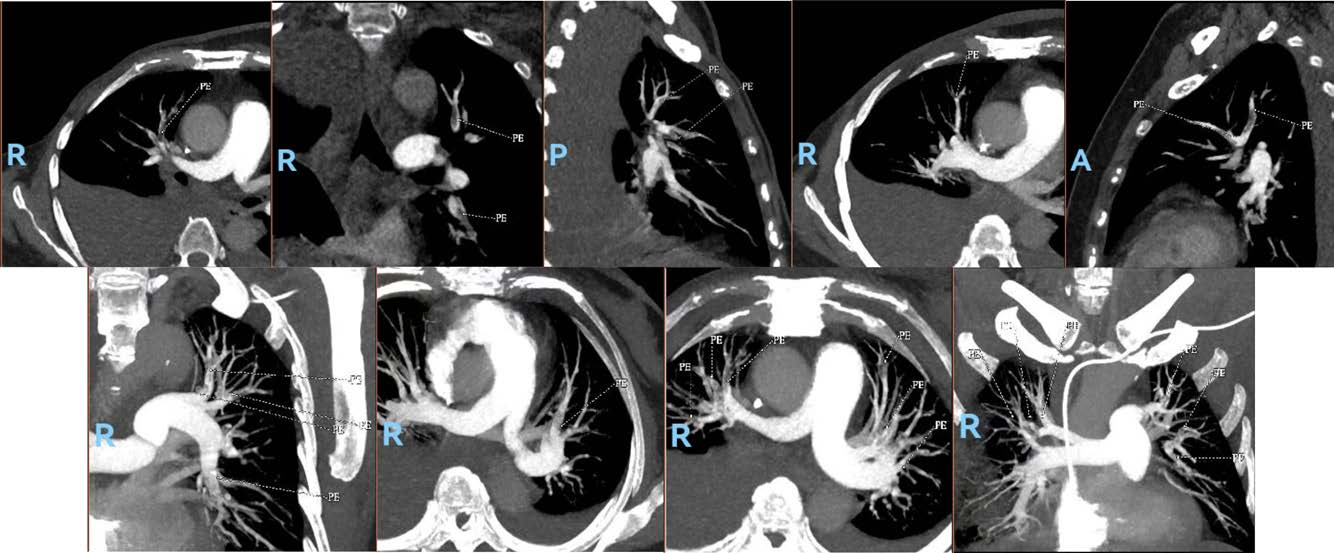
CTPA showed multiple emboli in both pulmonary arteries. CTPA = computed tomography pulmonary angiography.
